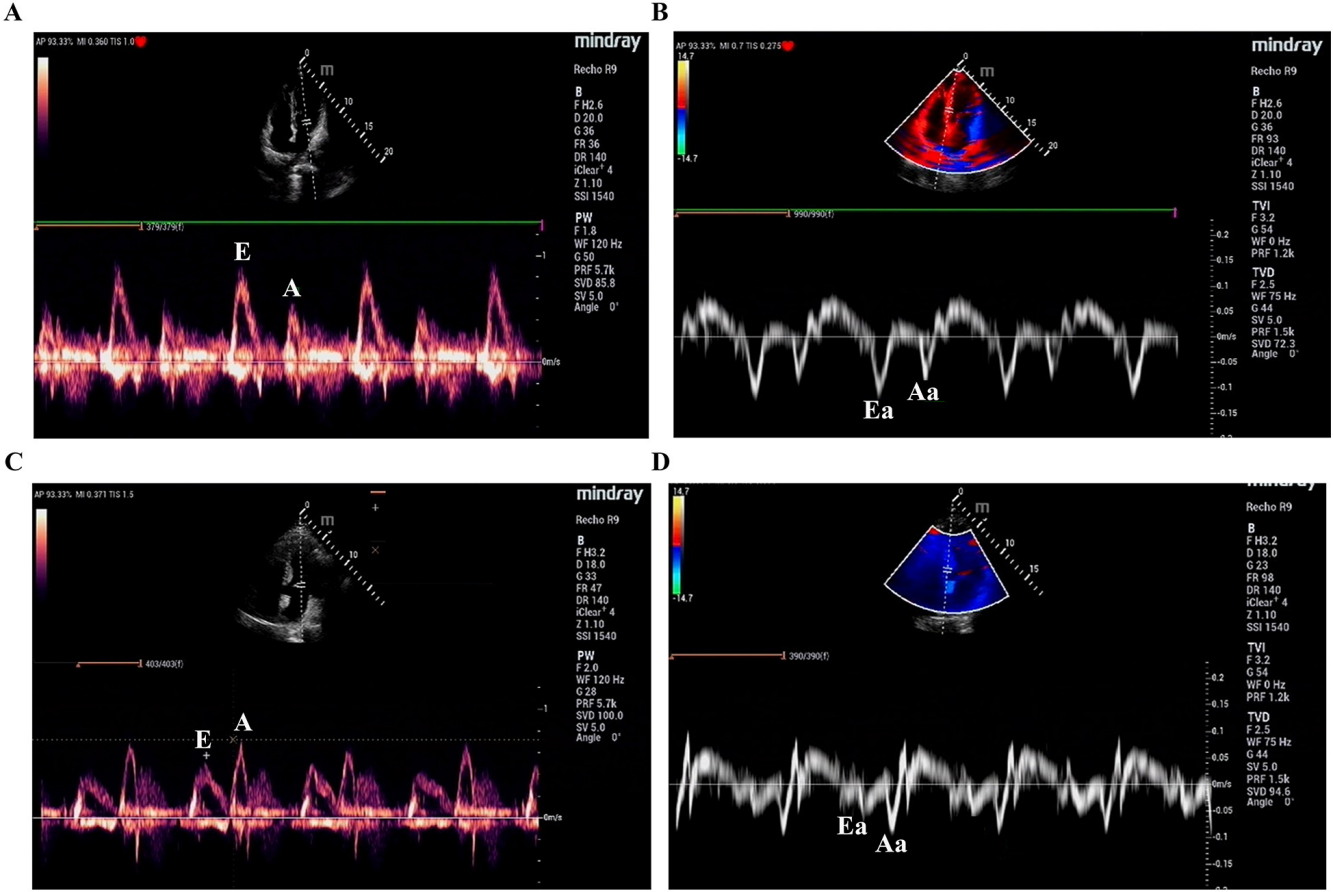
The pulsed Doppler (A,C)/tissue Doppler (B,D) imaging. Early mitral inflow velocity (E); Late mitral inflow velocity (A); Eearly diastolic myocardial relaxation velocity (Ea); Late diastolic velocity (Aa).
